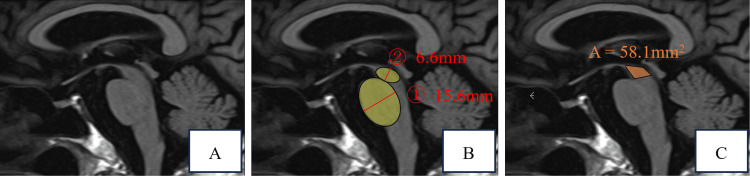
Sagittal T1 image on MRI (A) A hummingbird sign was seen because of the atrophy of the mesencephalic tegmentum. This sign is a characteristic MRI finding in PSP. It is observed in sagittal head MRI when the midbrain tegmentum atrophies. (B) ① Anterior-posterior midbrain diameter　② Anterior-posterior pons diameter Elliptical regions of interest were placed over the pons and the midbrain in the midsagittal slice. The maximal measurement perpendicular to the major axis was taken. The posterior border of the pons was clearly identifiable and did not include the pontine tegmentum. The midbrain measurement did not include the collicular plate. (C) Midbrain area A  straight line is drawn from the lowest part of the interpeduncular fossa to the lowest part of the corpora quadrigemina. This line is the boundary between the midbrain and the pons.
